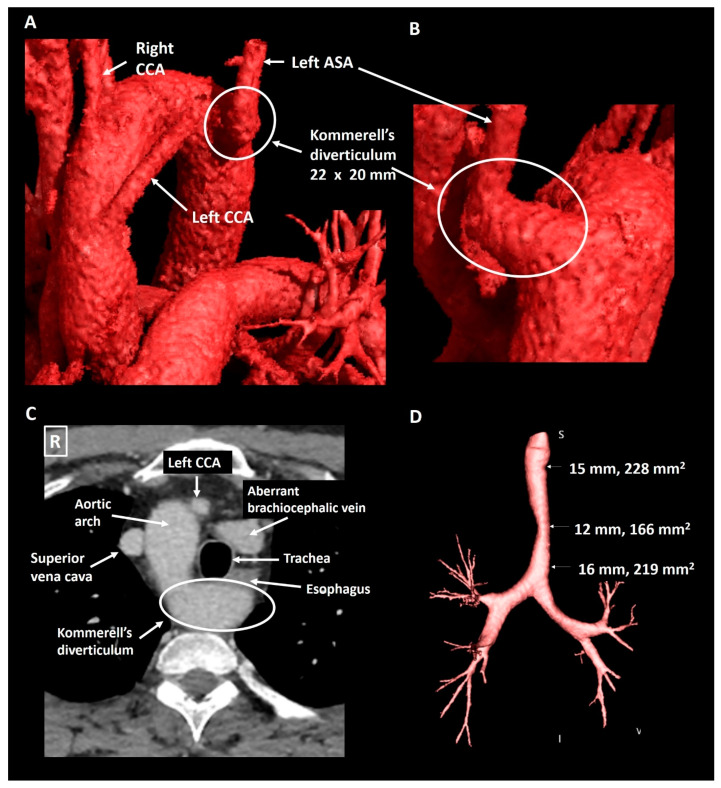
Patient 1. Panel (A) shows a CT-image reconstructed with global illumination rendering (iGIR) (Vitrea^®^, Vital Images) in a anterolateral orientation depicting a right-sided aortic arch with an aberrant left subclavian artery. Panel (B) shows a close up of the oblique posterior view of a Kommerell’s diverticulum as the onset of the aberrant left subclavian artery. Panel (C) is an axial reconstruction depicting the anatomical relationship between the aortic arch, Kommerell’s diverticulum and an aberrant brachiocephalic vein which encircle the trachea resulting in mild compression. Panel (D) is a minimum intensity projection of the trachea showing mild external compression. ASA, aberrant subclavian artery; CCA, common carotid artery.
